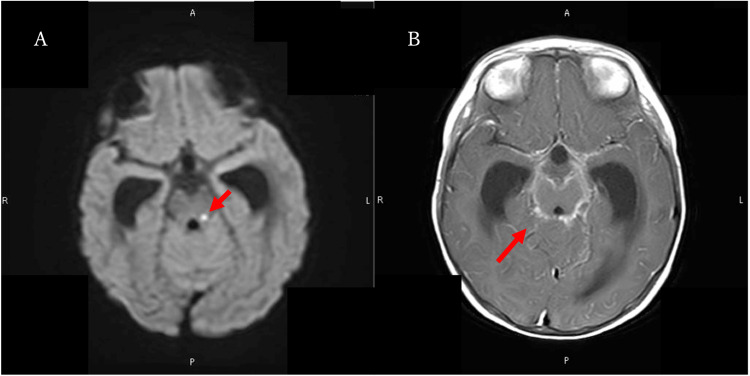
Brain magnetic resonance imaging performed at admission. (A) Diffusion-weighted imaging demonstrating infarction in the midbrain (arrow). (B) Contrast-enhanced T1-weighted magnetic resonance imaging showing enhancement along the brainstem in the basilar cisterns and sulci (arrow)
